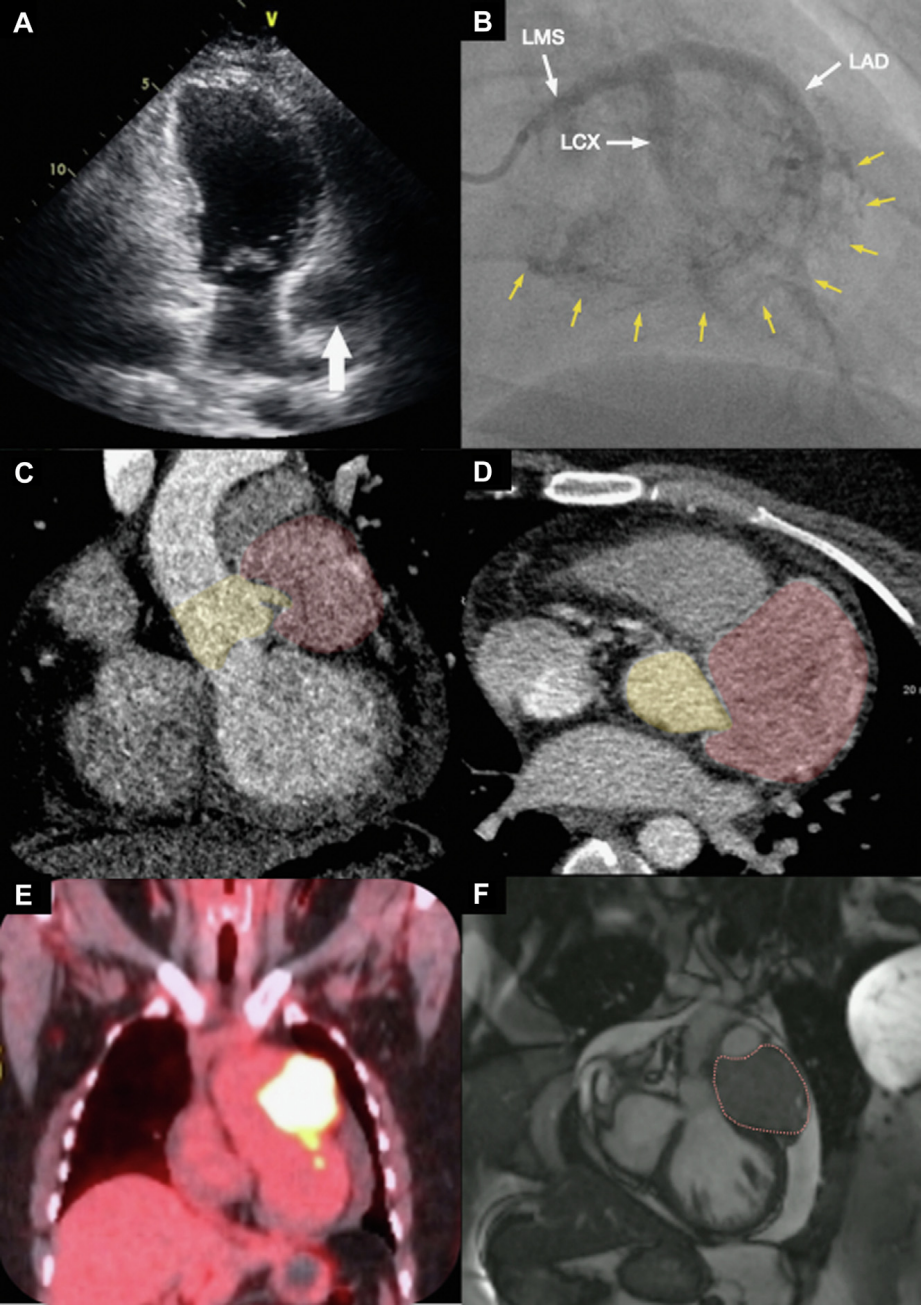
Multimodality Imaging Findings (A) Transthoracic echocardiogram shows an unusual appearance next to the left atrium (arrow) that is suggestive of extrinsic compression. (B) Coronary angiography demonstrating significant contrast blush (yellow arrows) from the highly vascular paraganglioma encasing the left coronary artery and its branches (white arrows). (C and D) Computed tomography thorax coronal and axial images of the coronary arteries showing the mass encasing and compressing the left main stem (LMS) and proximal left anterior descending coronary artery (LAD) (red: mass; yellow: aortic root and LMS). (E) Intense fluorodeoxyglucose F 18 uptake on positron emission tomography–computed tomography. (F) Dashes outline the intrapericardial paraganglioma on cardiac magnetic resonance imaging. LCX = left circumflex coronary artery.
